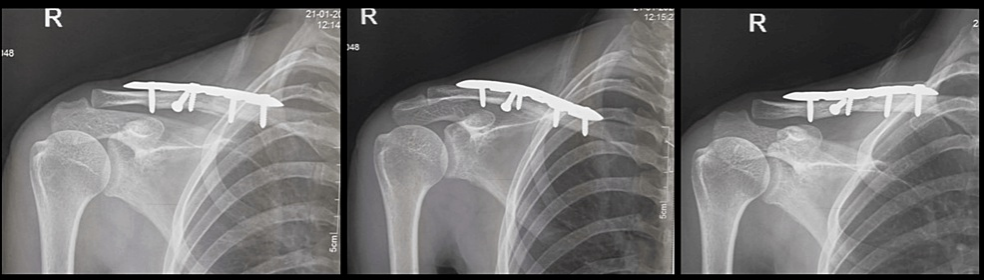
Post-operative clavicle fracture - AP, cephalic, and caudal X-ray views
